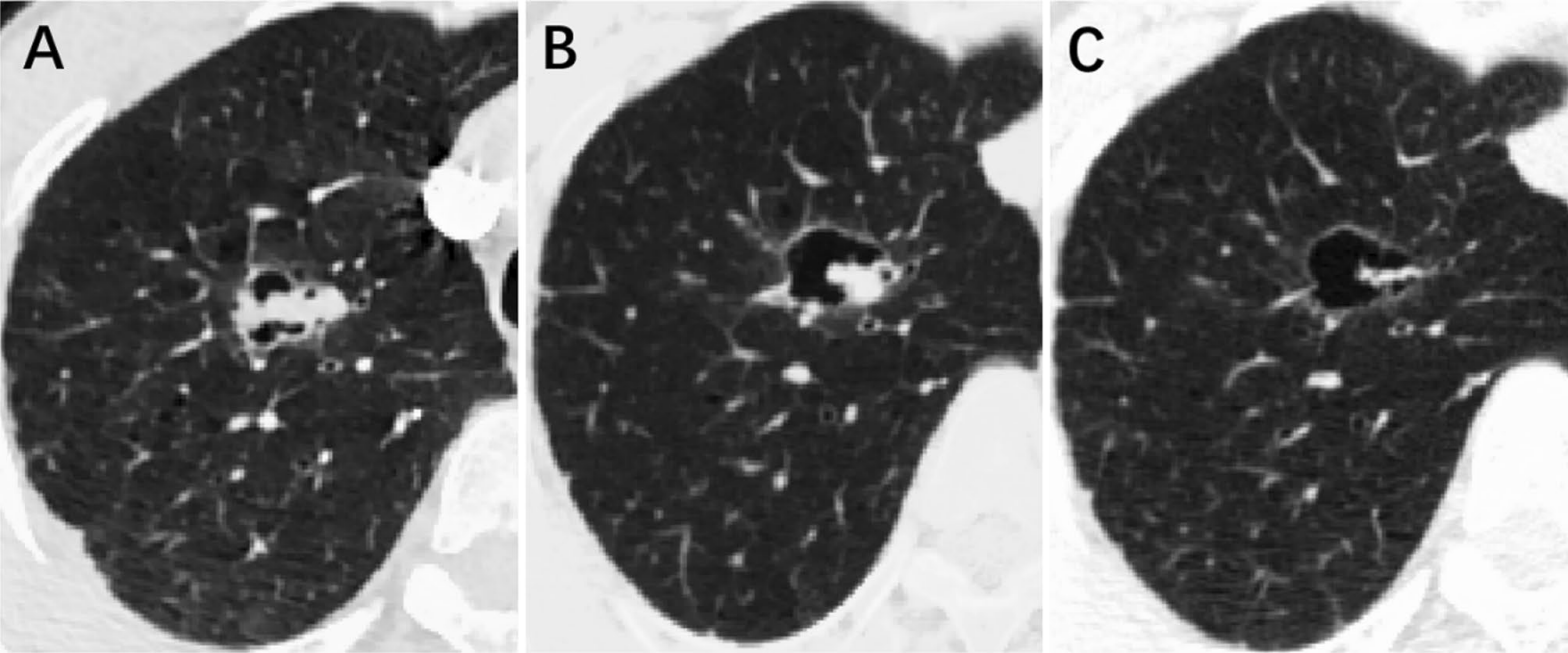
A 59-year-old male with lung cancer associated with cystic airspace (SSC). The lesion was located in the upper lobe of the right lung. (A) Axial-baseline CT imaging (January 21, 2020) showed solid-component and cystic-airspace components (type II). (B) The diameter of the solid component showed a significant decrease at the first examination during NC (April 24, 2020), while the cystic component was slightly enlarged. (C) The solid component continually decreased, and the lesion mainly manifested the cystic-airspace component at the last examination after NC (May 13, 2020).
